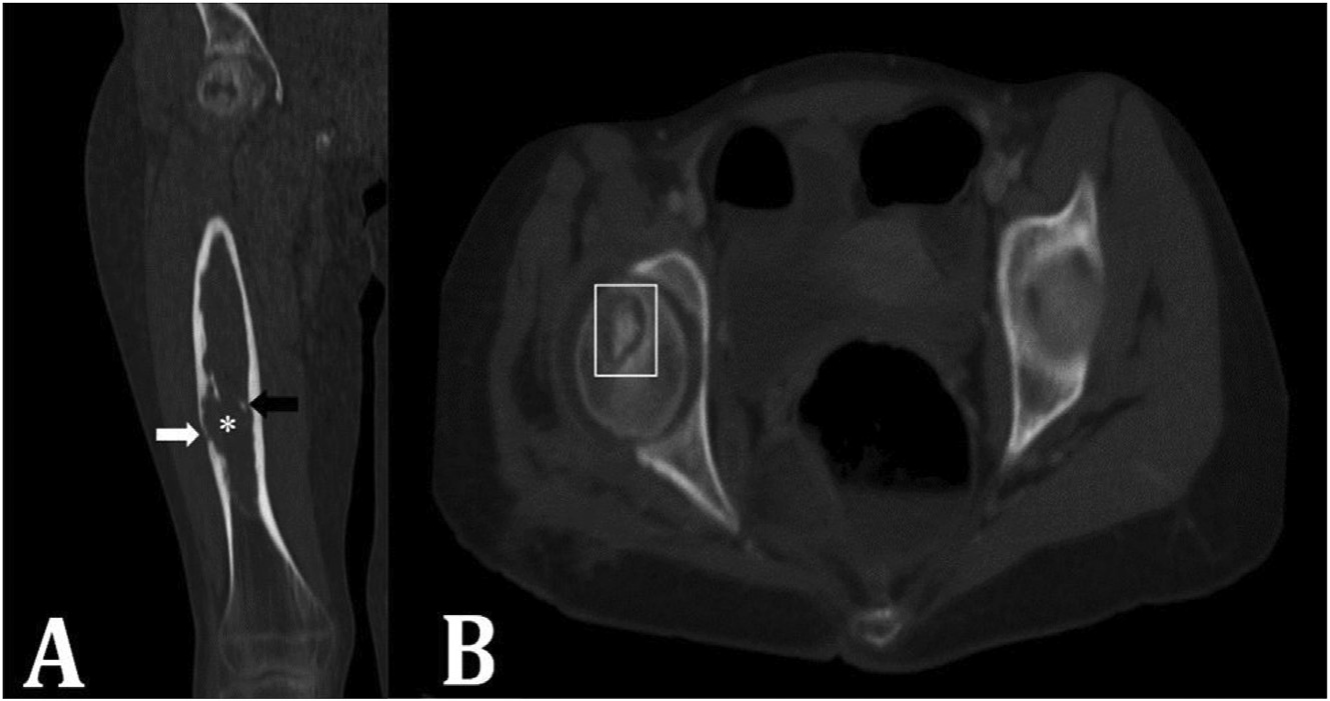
A) Coronal reformatted CT scan of lower limb in a 14-year-old girl with generalized hydatidosis shows expansile intra-osseous lesion (Asterisk) in the right femur. Note the areas of cortical thinning (white arrow) and intralesional calcifications (black arrow). B) Axial CT scan of pelvic bones shows a lytic lesion in the femoral head with internal calcifications (rectangle). Note the intra-articular involvement. (Photos are from our own patient(s) and they had not been published previously elsewhere.).
